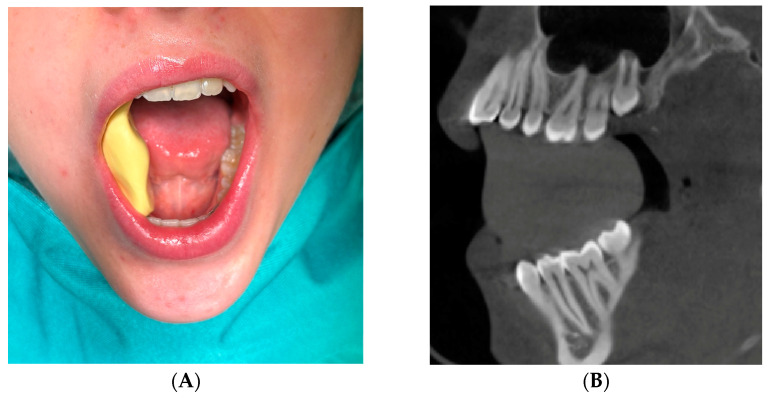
(A) Intraoperative view of the patient with the bite block in place before surgery; (B) Cone beam computed tomography image in the sagittal plane displaying the bite block that stabilizes the mandible in a standardized position, eliminating visualization errors due to jaw movement.
